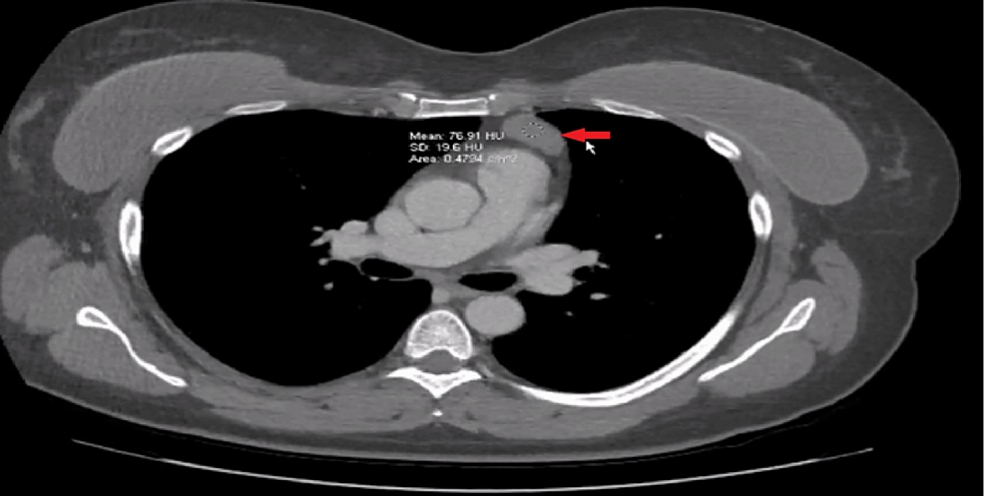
CT of chest showing thymoma (red arrow). CT, computerized tomography
